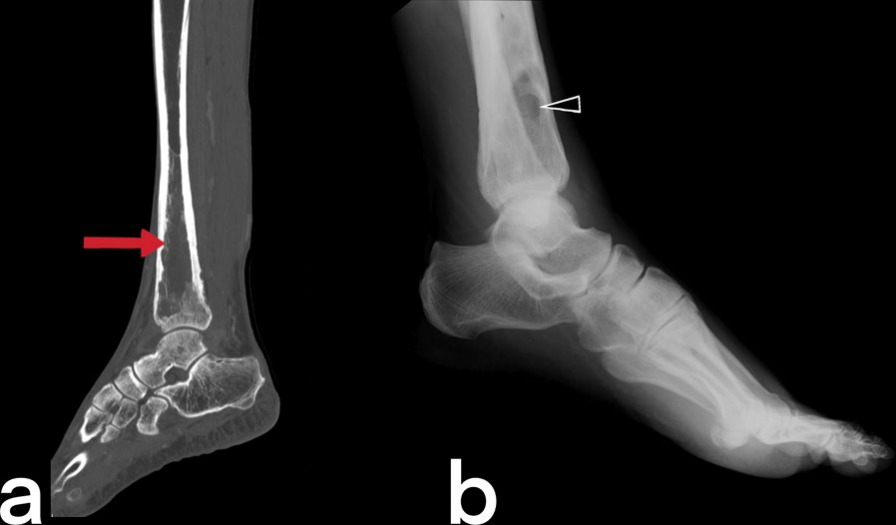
CT (a) and lateral ankle direct X-ray (b) images of two different patients. Lytic areas are observed in the tibia in both cases with proven tuberculous osteomyelitis and abscess
